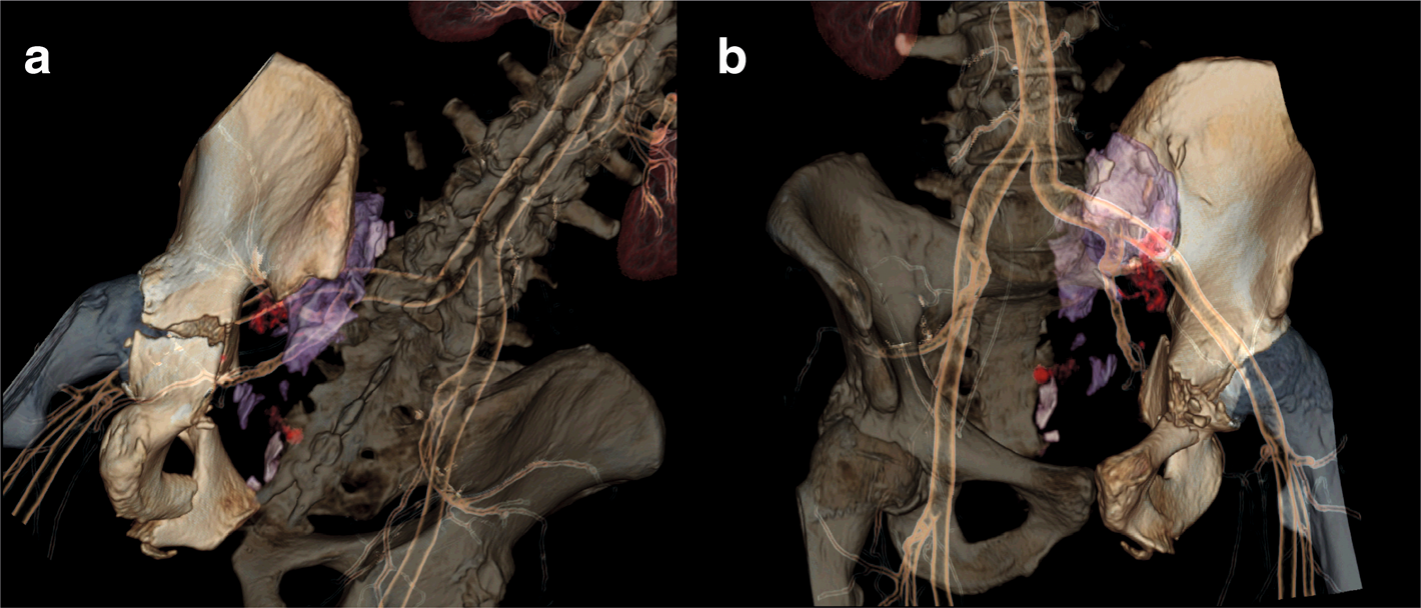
a and b: *3D Volume rendering*. 3D volume rendering reconstructions of the initial CT scan prior to endovascular intervention in an anterior (a) and a posterior (b) view. Central acetabular dislocation fracture on the left side (OTA 62.B1.1), and unstable pelvic fracture (OTA 63.C1.3) with vertical sacral fracture and anterior pelvic ring disruption. Disruptions of the left superior gluteal and a sacral arteries with large extravasation of contrast material
